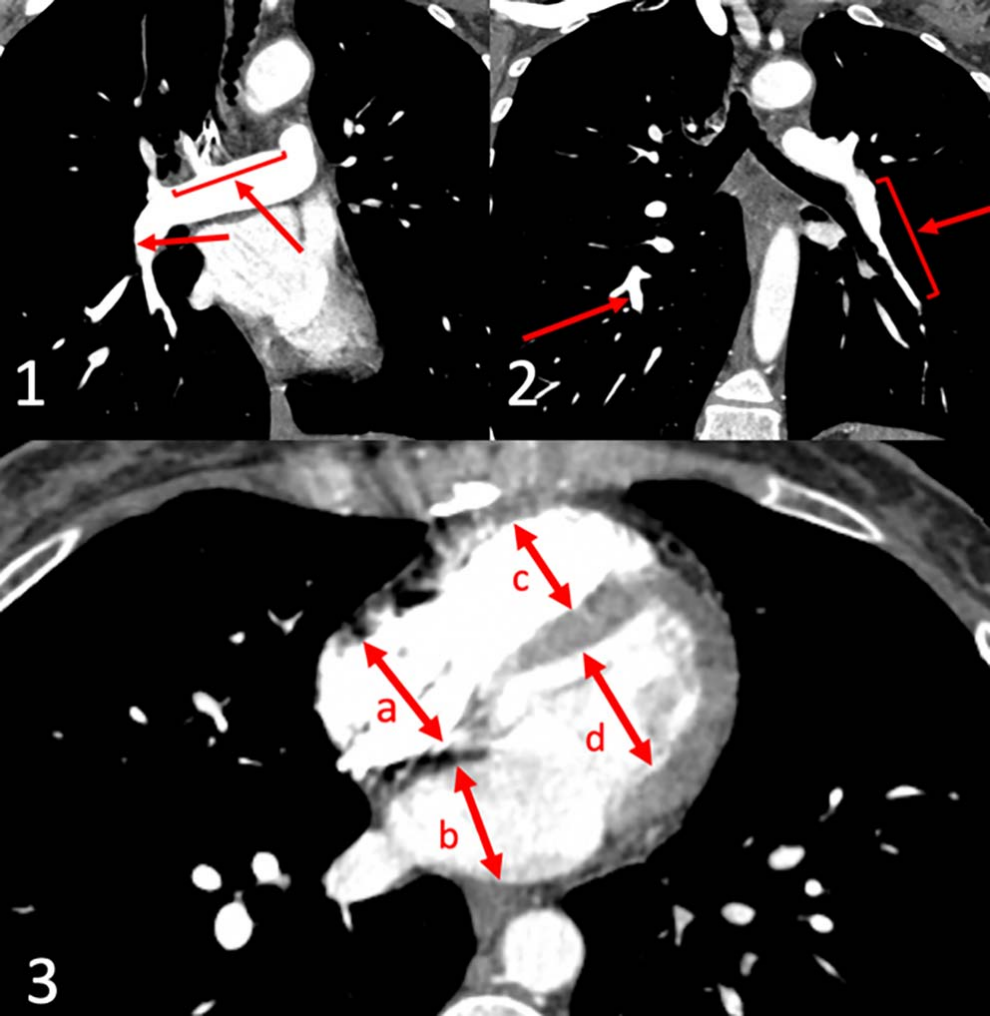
CT angiogram 6 months after discharge: 1 – coronal view shows absence of thrombi after pulmonary endarterectomy in right main and left inferior lobar pulmonary artery (red arrows); 2 – coronal view shows absence of thrombi after pulmonary endarterectomy in left inferior and right inferior lobar pulmonary arteries (red arrows); 3 – axial view shows right atrium (a) and right ventricle (c) remodeling after surgery compare to left atrium (b) and left ventricle (d).
